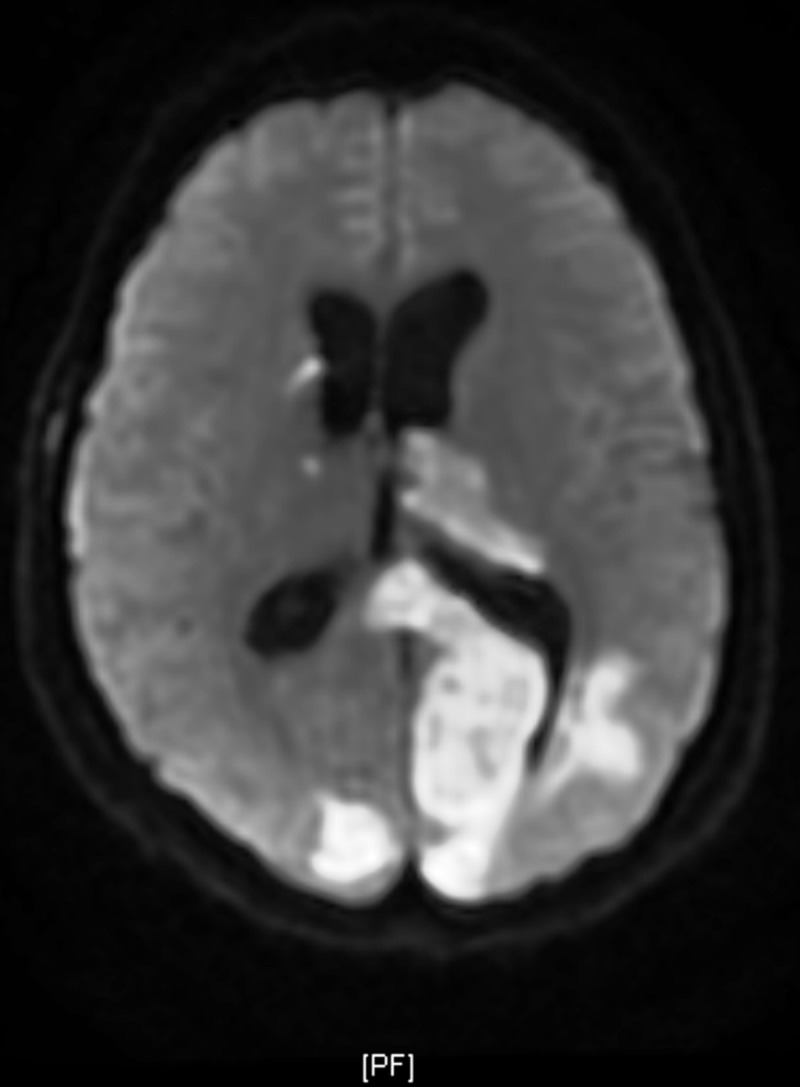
Magnetic resonance imaging (MRI) images diffusion-weighted imaging/apparent diffusion coefficient (DWI/ADC) showing an acute infarct in the left occipitoparietal, left thalamic, and right occipital region involving both the posterior cerebral artery (PCA) territories.
